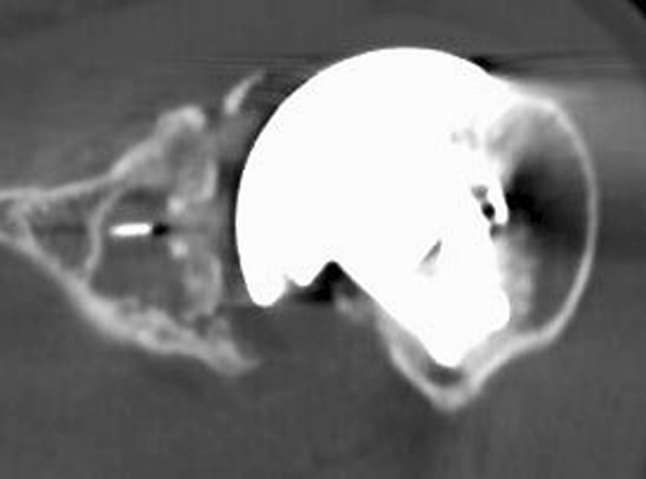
CT scan evaluation: no tissue integration around the central peg
